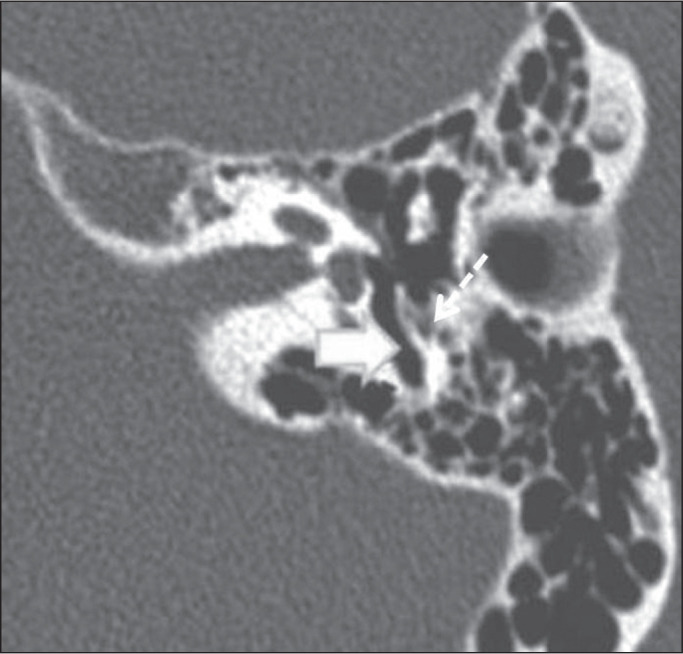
Deep sinus tympani. Unenhanced axial CT of the temporal bone, showing
